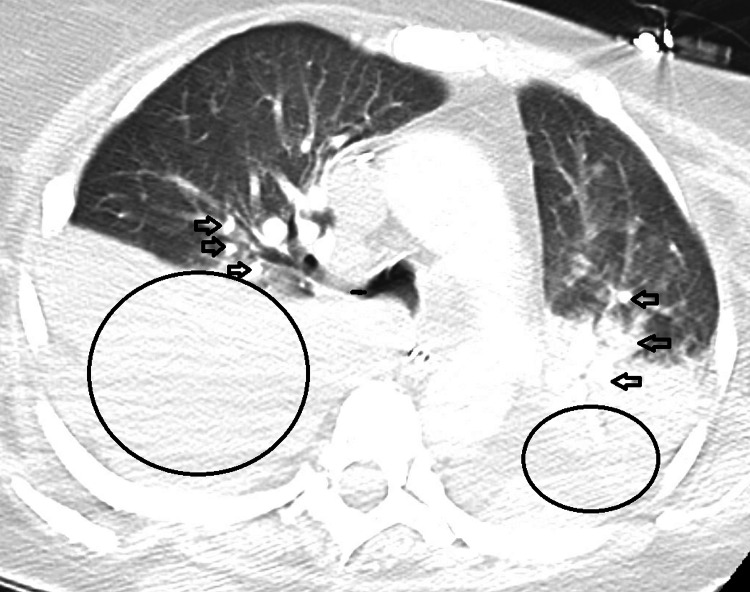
Computed tomography scan of the chest. Black circles: Bilateral pleural effusions were identified, right side significantly greater than the left. Black arrows: Areas of nodular hyperdensities are recognized bilaterally with lobular infiltration evident as well.
